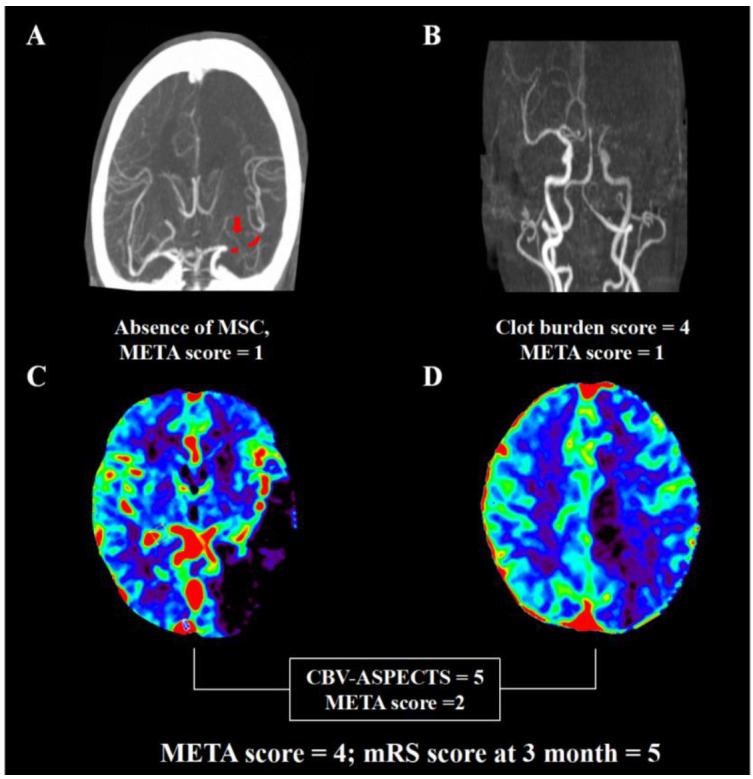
Representative images for calculation of META. A male patient had occlusion of left middle cerebral artery on dynamic computed tomography angiography (A,B). He had clot burden score of 4 (occlusion of M1 and M2), one segment of clot (red dot that the red arrow points to, (A)), good collateral with >50% filling of the occluded middle cerebral artery territory (A). Cerebral blood volume map (C,D) demonstrated the Alberta Stroke Program early CT score of 5. Thus, the META score was 4. The modified Rankin score was 5 at 3 months despite successful recanalization.
